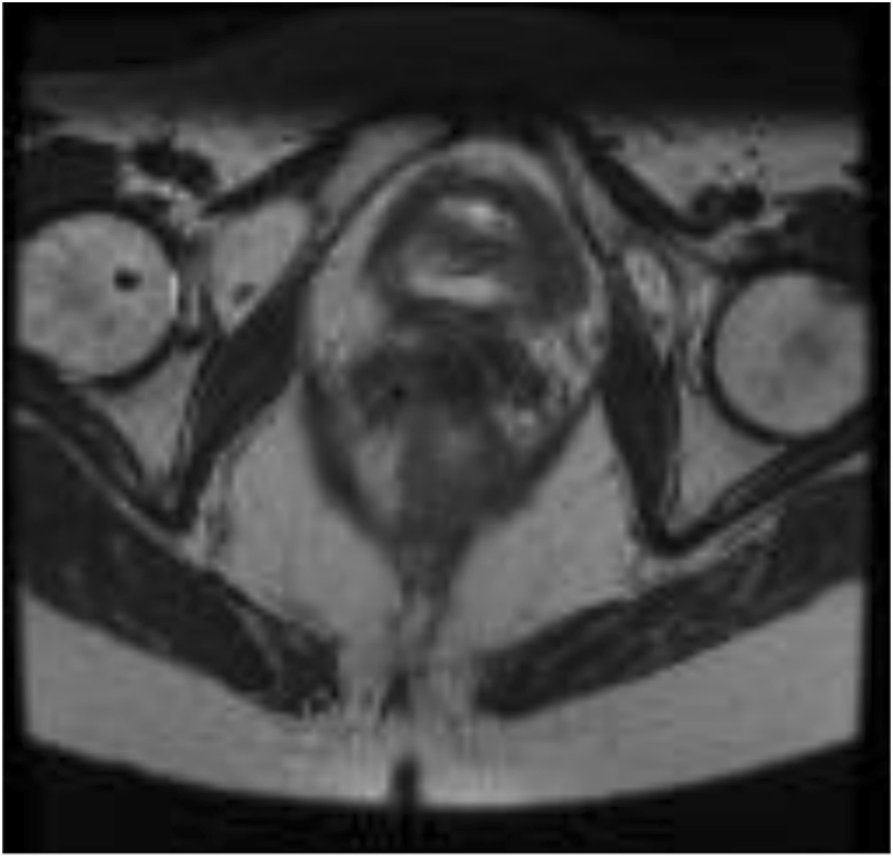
Transverse T2 MRI of the patient with uterus bicornis and bicollis and two cervical ossa.
